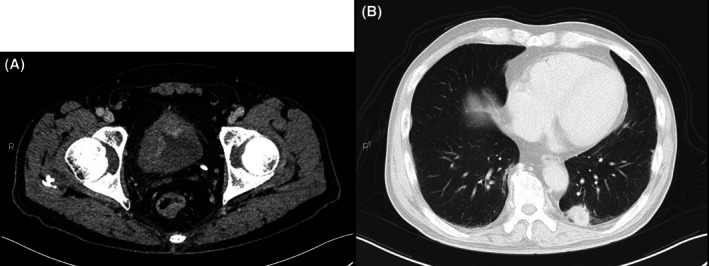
CT findings. (A) Nodule in the left pulmonary. (B) Multiple bladder tumors.
